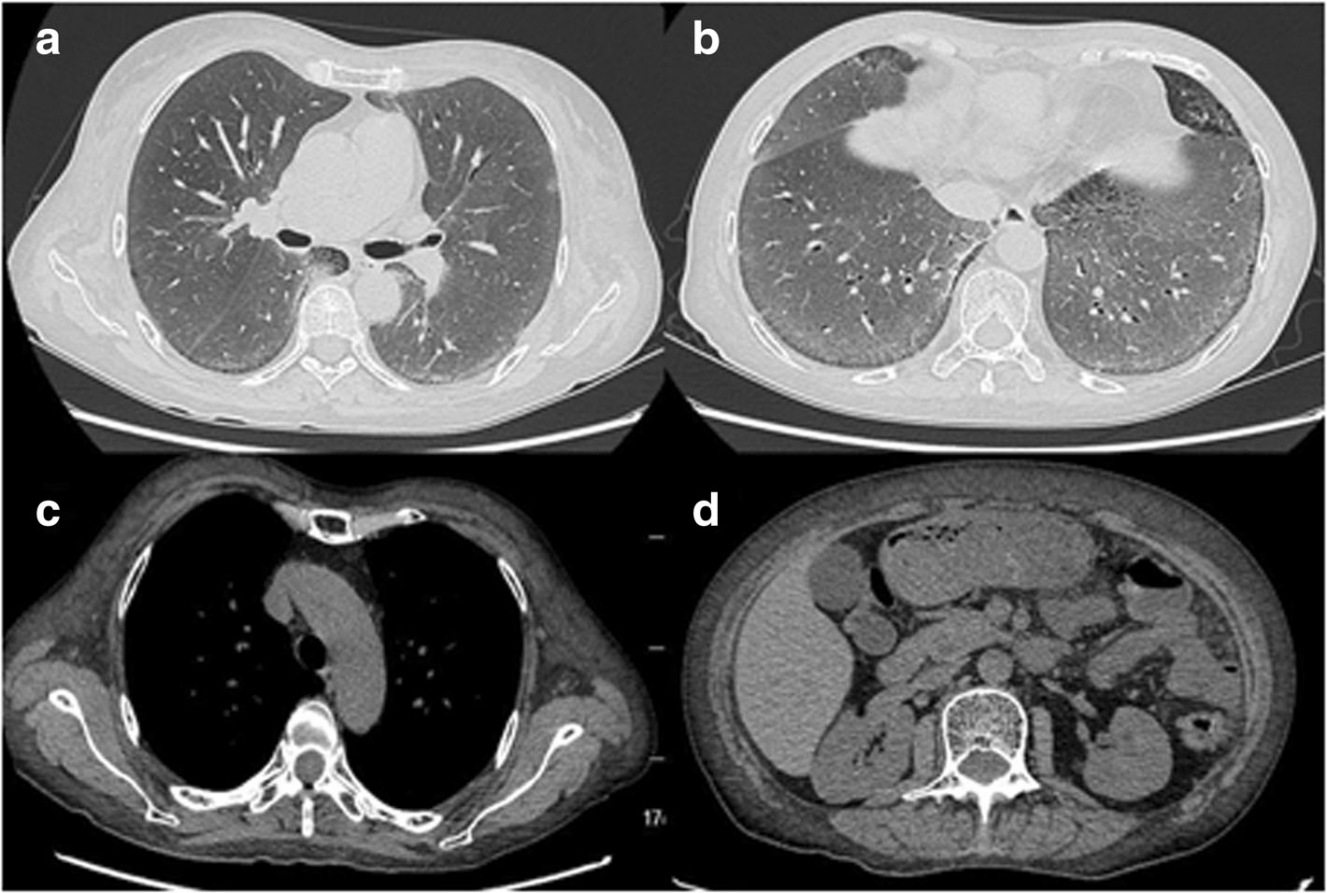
Chest and abdominal CT. (a, b) showed ground-glass opacities with interlobular septal thickening in bilateral lungs. (c, d) showed soft tissue infiltration of the subcutaneous fat layer with asymmetric bulging of the chest and abdominal wall
